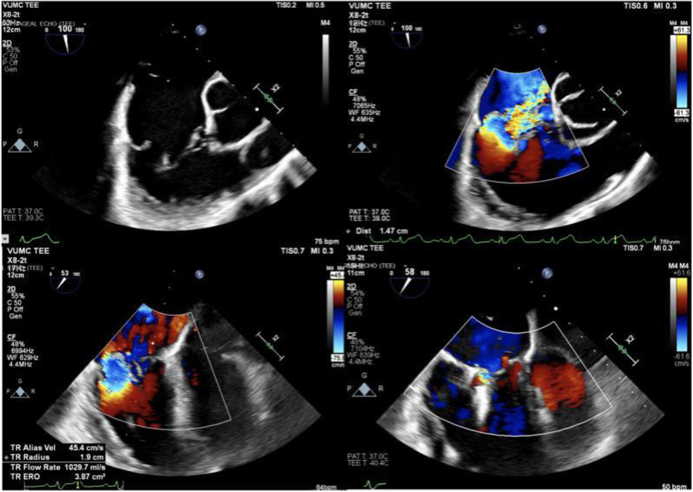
Transesophageal echocardiography showing TriClip tricuspid valve repair. Transesophageal echocardiography image demonstrating TriClip tricuspid valve repair. Tricuspid regurgitation improved from torrential to trace after placement of an anterior-posterior leaflet device and a second device in the posterior-septal location. Source: Authors’ Creations.
